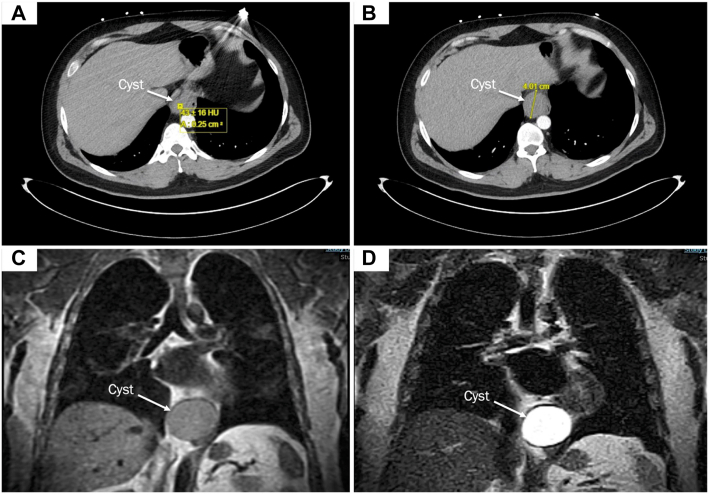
Imaging of the mass. Computed tomographic angiogram showing a paraesophageal mass with (A) 43 Hounsfield units (HU) and (B) measuring 4 cm. On magnetic resonance imaging, the mass appears (C) dark on T1-weighted imaging and (D) bright on T2-weighted imaging (D), findings suggesting that the mass is fluid filled.
